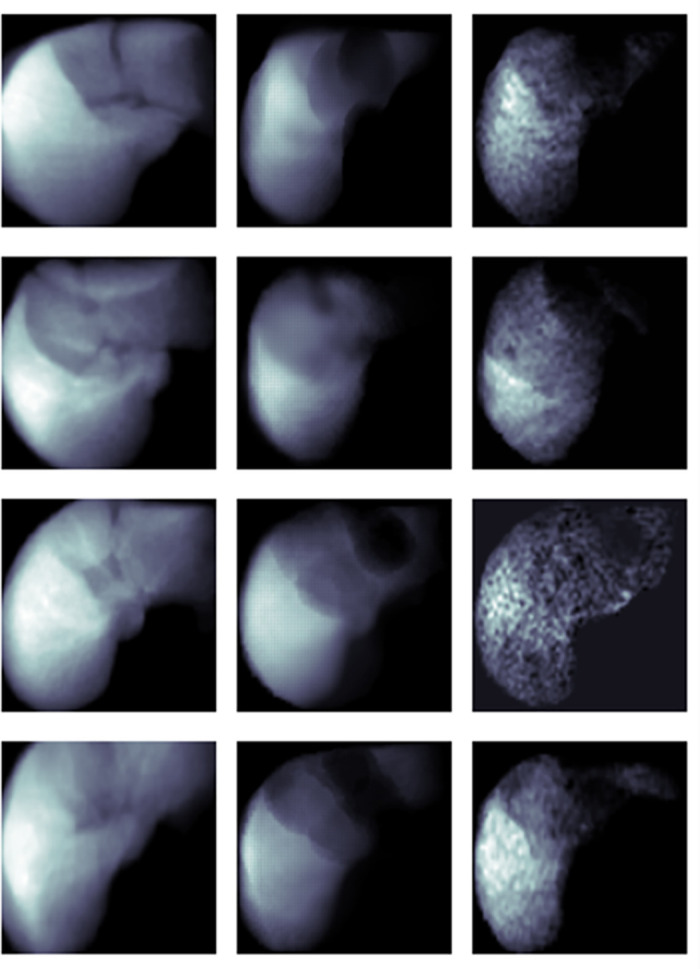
Average intensity projection (AIP) of the 3D volumes: Input CT image (left), PDFF inference as model output (middle), reference PDFF images (right).
